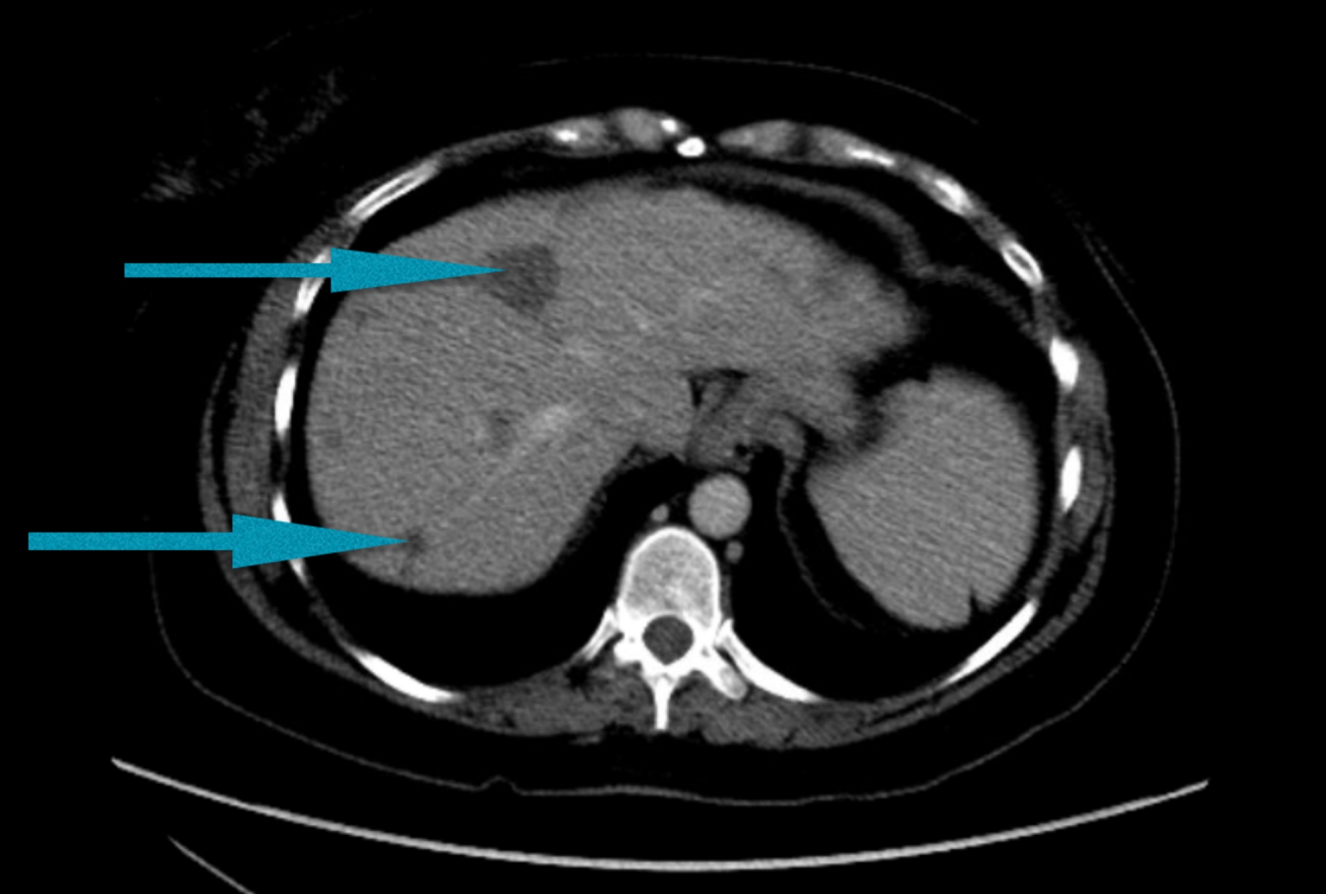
Multiple metastases to the liver shown by blue arrowheads on a computed tomography scan of the abdomen and pelvis
